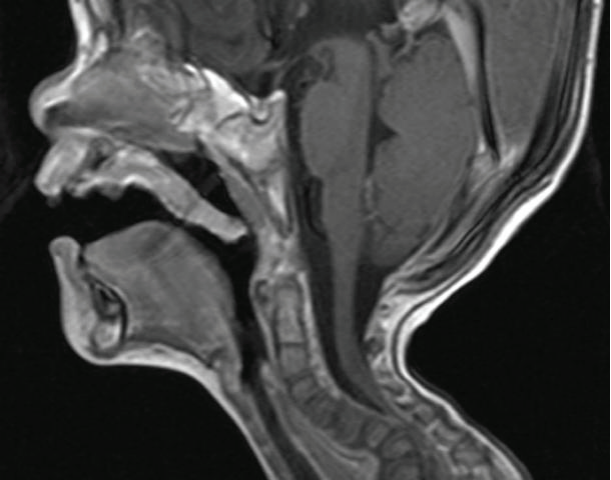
T2-weighted magnetic resonance imaging sequence that was made post intervention, showed severe impingement on the spinal cord at the level of C7. Dramatic progression of the kyphosis to 90 degrees had occurred. In addition significant hypoplasia of the cervical vertebral bodies along the spine segments C5/7.
